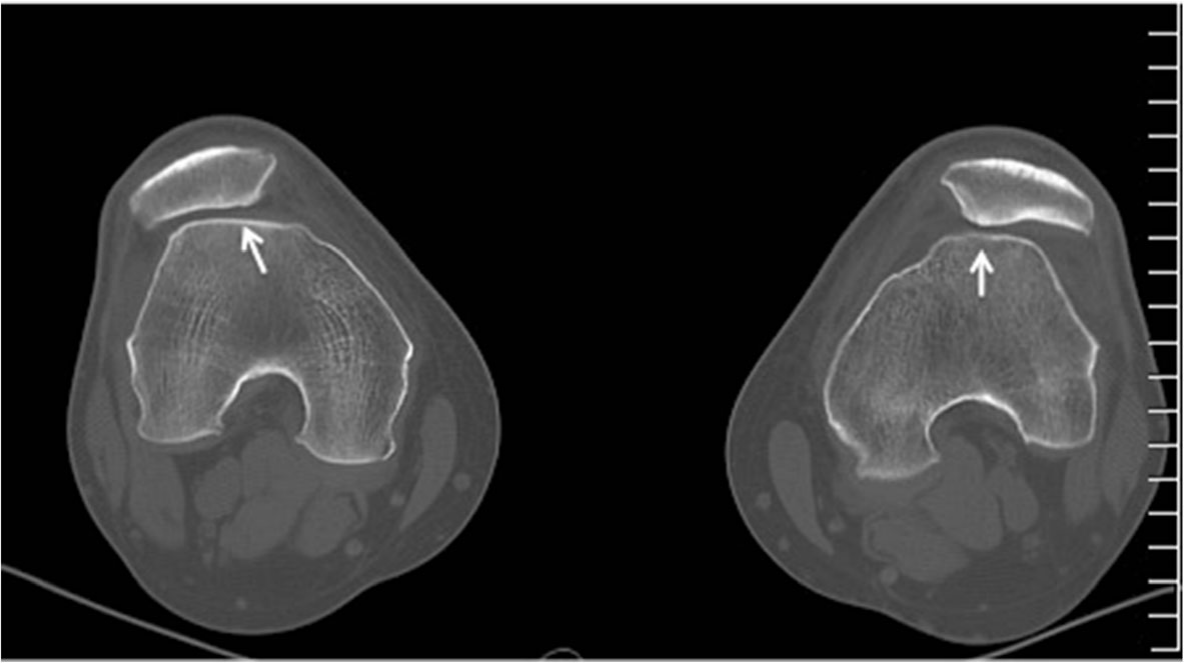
CT of both knees in 20° flexion demonstrating bilateral shallow trochlear groove (arrows) compatible with dysplasia and bilateral lateral patellar subluxation and lateral tilt. The two features are associated with patellar maltracking
